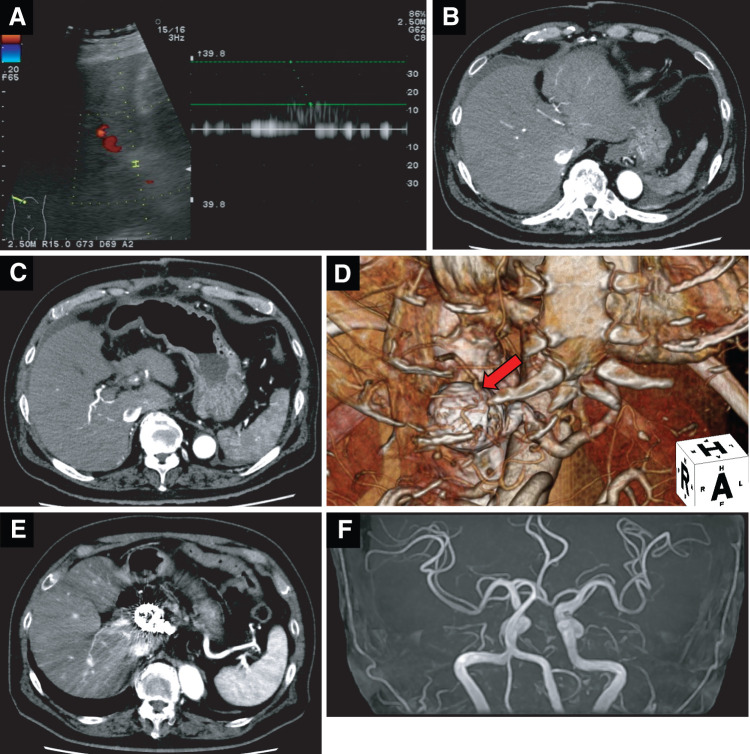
Postoperative confirmation of arterial flow, checking the regrowth of intraabdominal aneurysm, and excluding intracranial aneurysm. (A) Doppler US. (B and C) Enhanced CT on POD 4. (D) 3D reconstruction of arterial anastomosis between the MCA and LHA (red arrow: treated aneurysm). (E) Enhanced CT one and a half years after the operation. (F) MRA. LHA, left hepatic artery; MCA, middle colic artery; US, ultrasound
